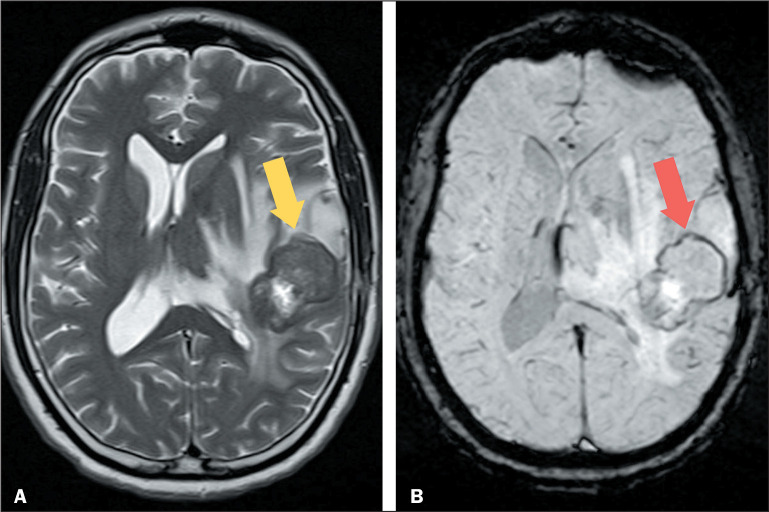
MRI of a patient with biopsy-confirmed cerebral
